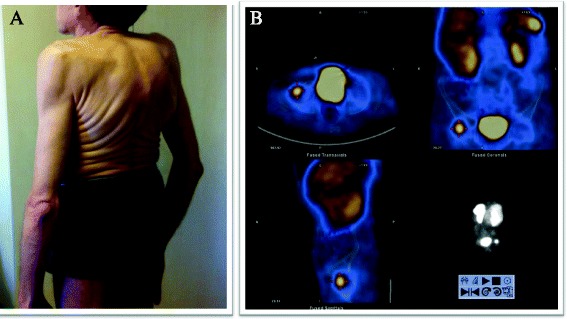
a The posture before the right hip endoprosthesis operation and tumour extirpation. The patient has given the written consent for his medical findings and photos to be published. b 99mTc-Tektrotyd whole body scintigraphy. Intensive, focal radiotracer uptake in right femur head (* red markers around*) (year 2014)
